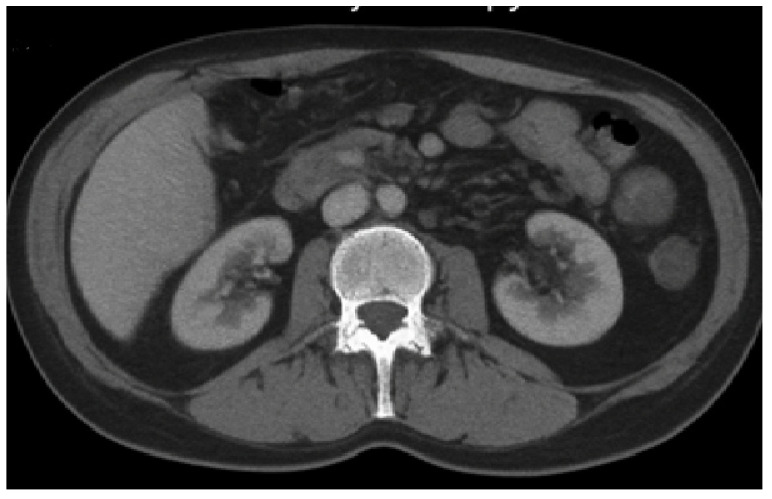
A contrast-enhanced CT scan of the abdomen and pelvis.
